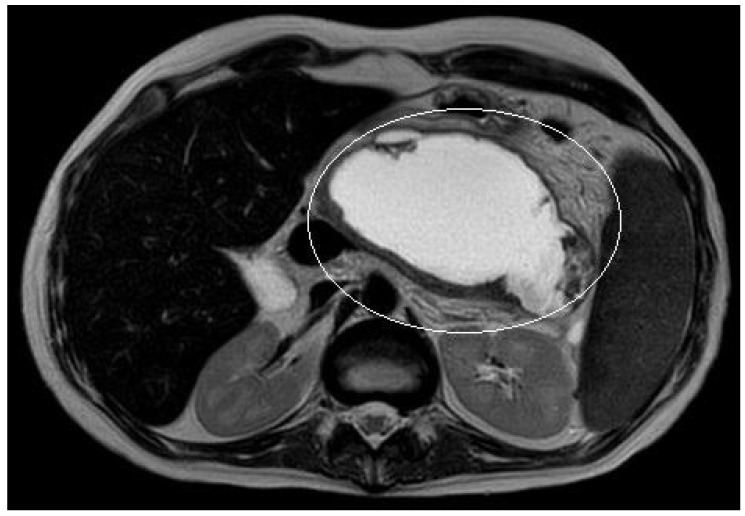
Patient n°2 MRI. Pseudocyst with maximum diameter 12 cm in the left hypochondrium in close proximity to the posterolateral wall of the stomach.
